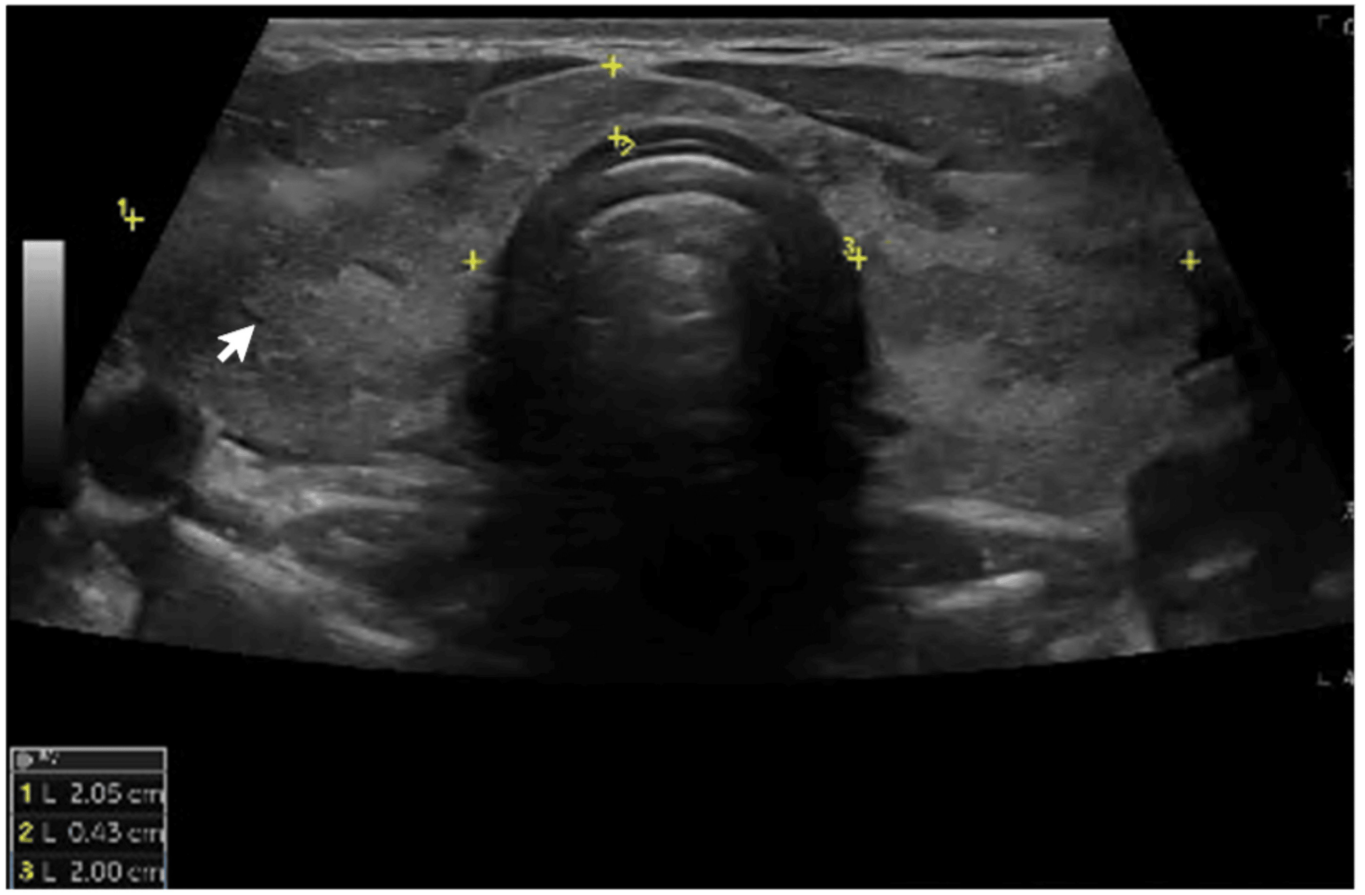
Thyroid ultrasound The ultrasound image demonstrates an enlarged thyroid gland with a diffusely hypoechoic texture (white arrow)
